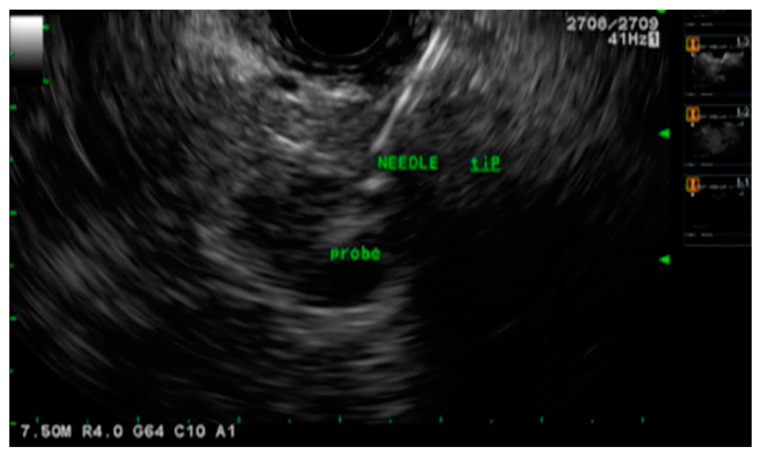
Endoscopic ultrasound-guided radiofrequency ablation.
